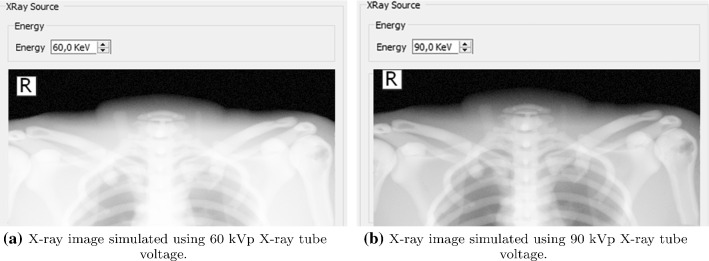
Effect of the X-ray tube voltage on an X-ray image of the chest
